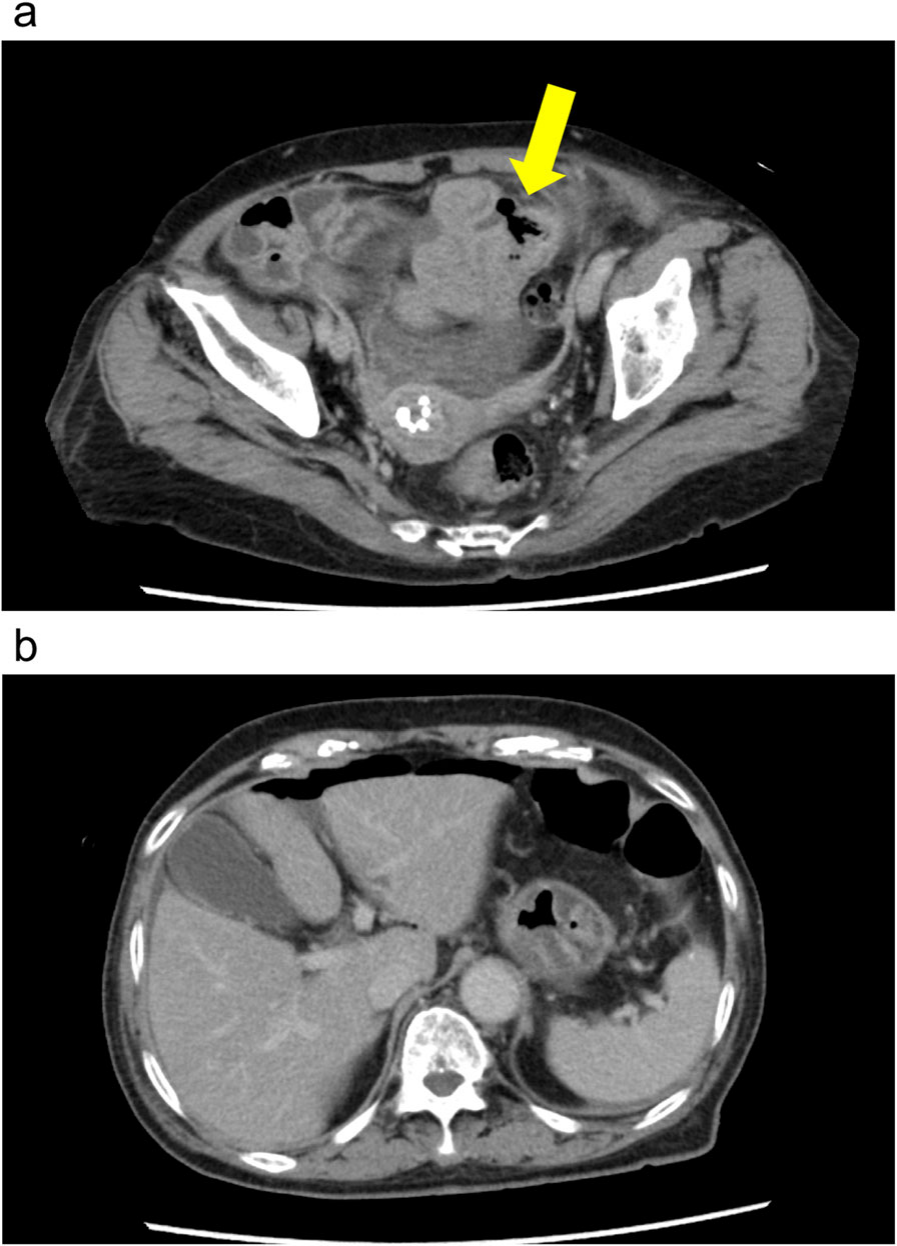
Representative images of CT scan on hospital day 3. a Perforation site (arrow) of the small intestine and abdominal effusion. b Free air in the abdomen. CT computed tomography
